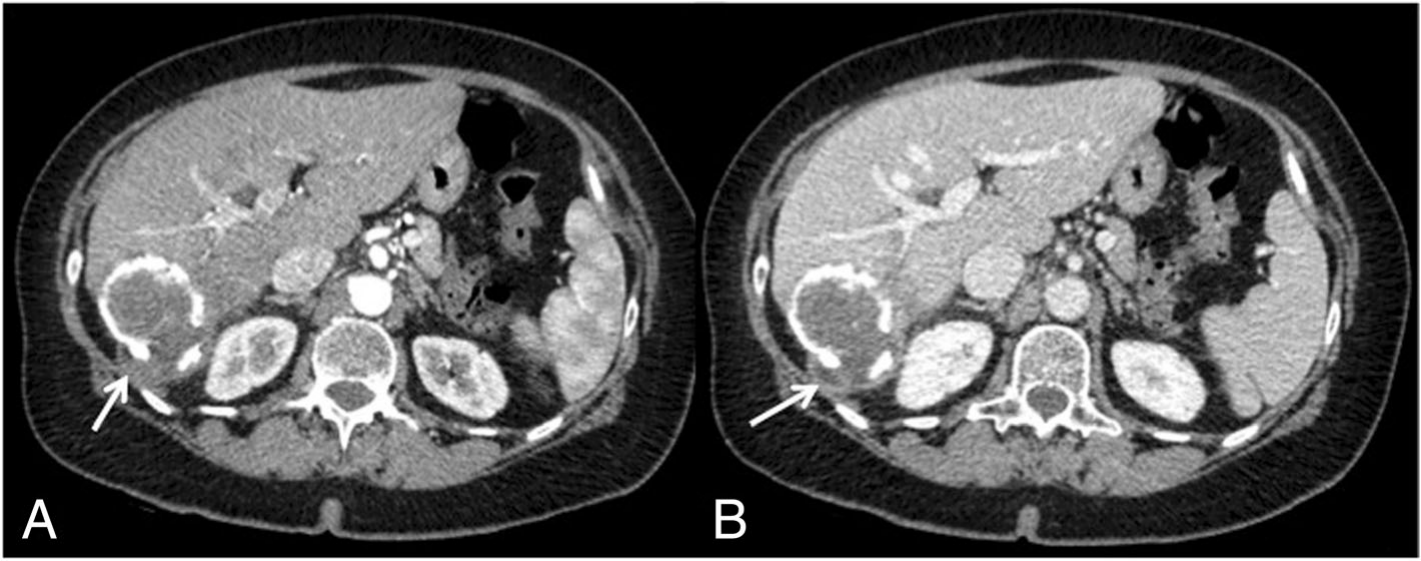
Axial CT view during arterial (a) and venous (b) phase. An incomplete wall is seen along the posterior side of the cyst in connection with an hypoattenuating area corresponding to extrahepatic extravasation of the cyst contents (arrows) due to extrahepatic rupture of the hydatid cyst
